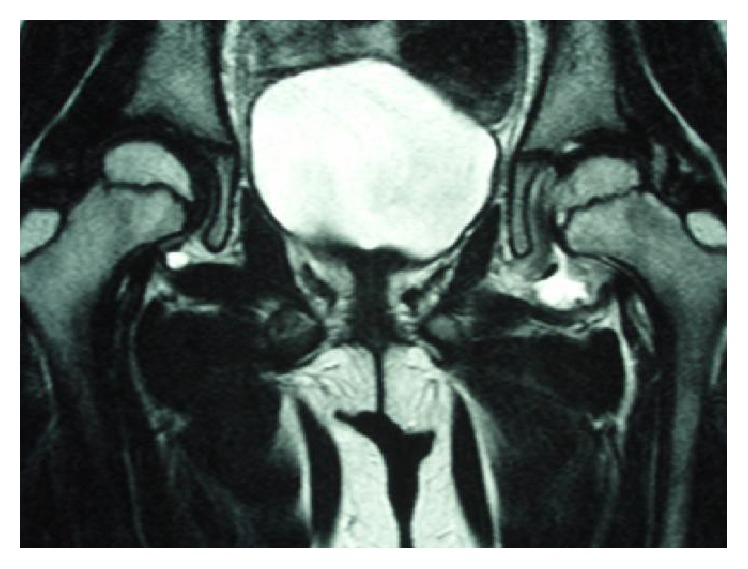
Coronal, T2-weighted magnetic resonance imaging scan of the hip joints 2 months after the initial visit. A linear low-signal-intensity region was found at the left femoral capital epiphysis.
